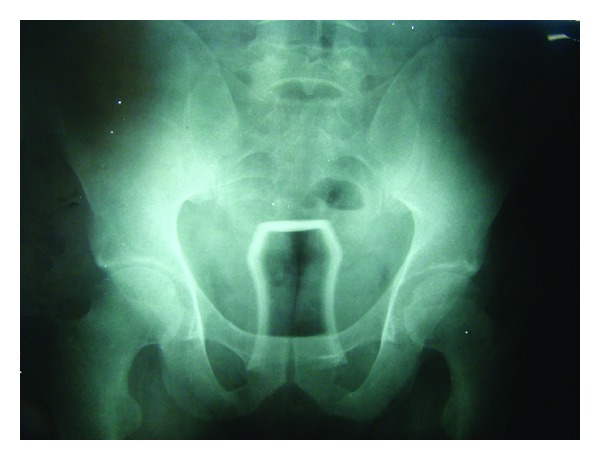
X-rays showed a glass inserted in the rectum.
